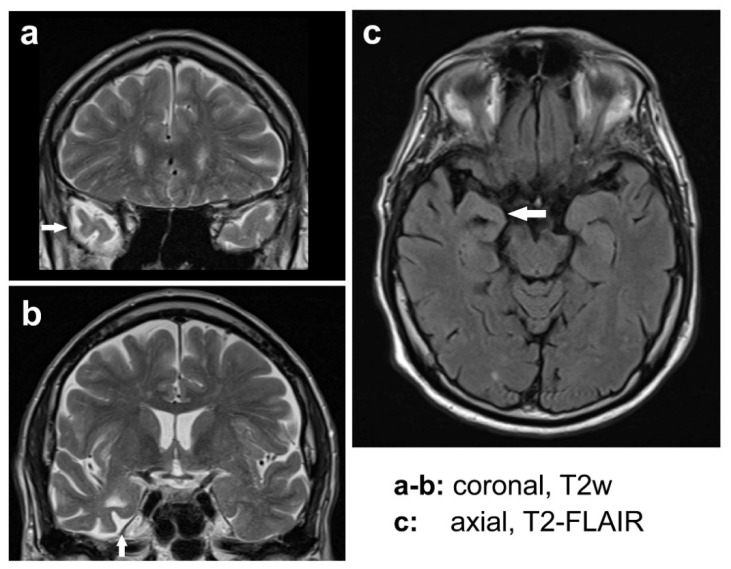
Magnetic resonance imaging showing right-sided medial temporal lobe atrophy (white arrows). Coronal plane, T2-weighted (T2w) turbo spin echo sequence (a,b); axial plane, T2-weighted fluid-attenuated inversion recovery sequence (T2-FLAIR) (c).
